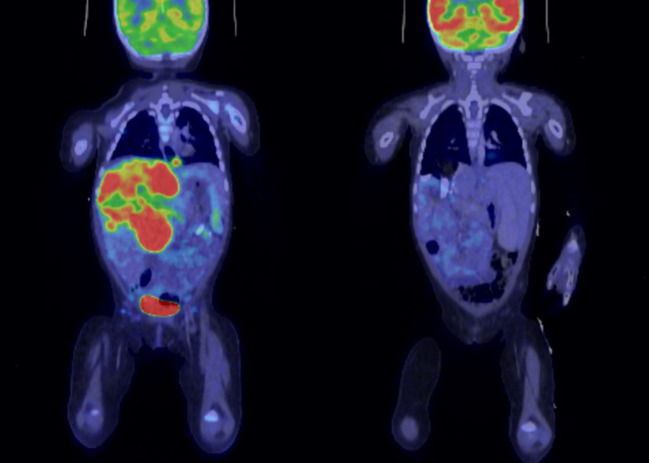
For patient P11 with extra-renal rhabdoid tumor (liver), fluorodeoxyglucose positron emission tomography (FDG-PET) (A) at diagnosis with FDG avid hepatic lesion; (B) status post hepatic lobe resection and eight cycles of VDCy/ICE showing response with small amount of residual FDG uptake at the surgical margins and no distinct mass identified.
